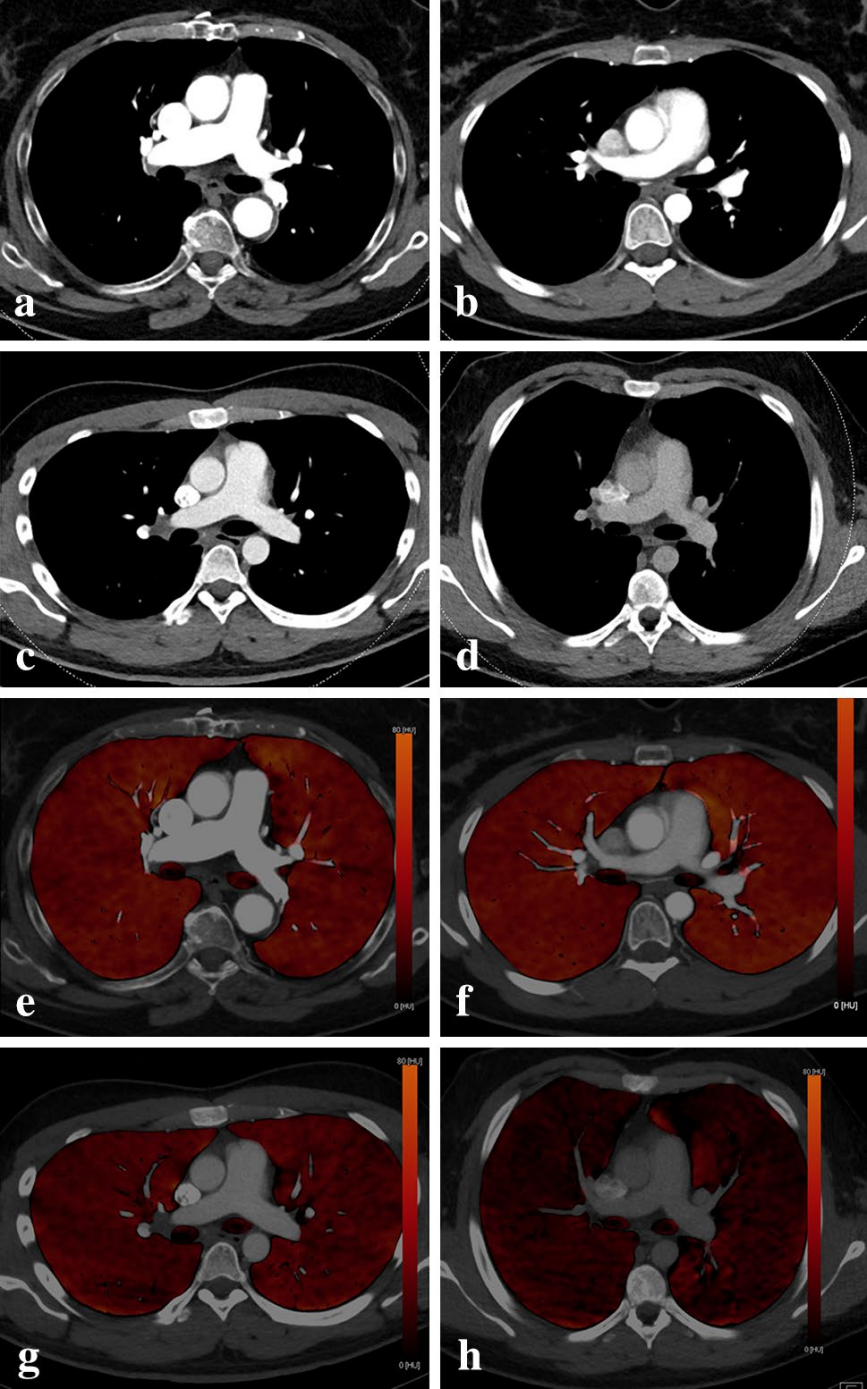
CT pulmonary angiography (a–d) and iodine map (e–h) with identical window settings for protocol A (a, e), B (b, f), C (c, g) and D (d, h). Note the decrease in pulmonary vascular contrast and iodine map enhancement from A to D
